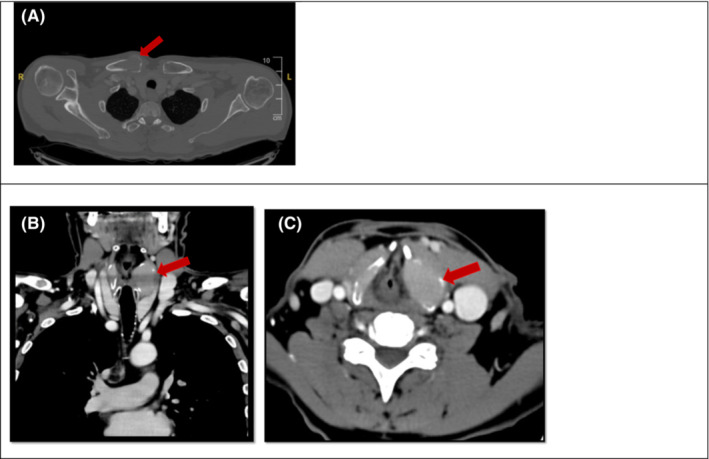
Multislice CT‐scan of neck and chest. (A) Osteolytic bone lesion in the medial right clavicula. (B,C) Multiple soft tissue density masses in the right and left thyroid cartilage. The largest is on the left with a locoregional mass effect (30 × 23 × 33 mm).
